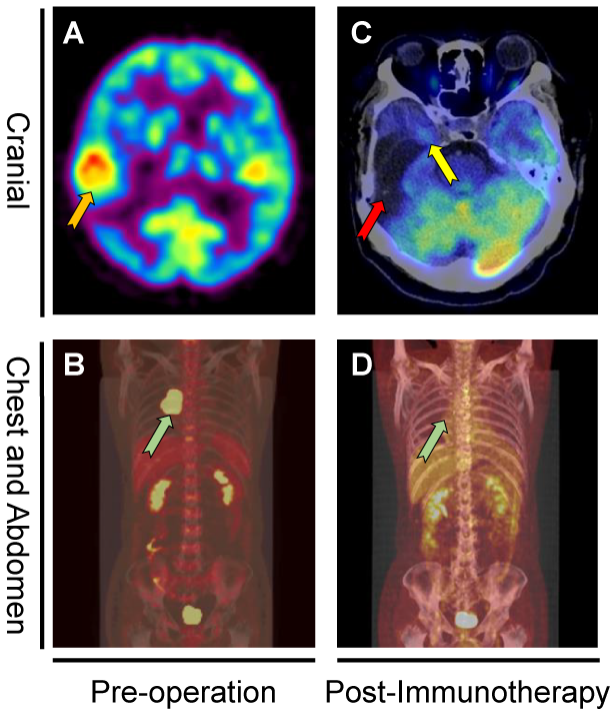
PET-CT before first cranial surgery and after immunotherapy. PET-CT showed a right temporal lesion (orange arrow) before the first cranial surgery (A). Coronal PET-CT image (B) of the chest showed a right mediastinal metastasis (green arrow). Figure (C) showed the original tumor sites (red arrow and yellow arrow) remained stable without diseases recurrence. Coronal PET-CT image (D) of the chest indicated the tumor site (green arrow) remained stable without recurrence 16 months after mediastinal surgery.
